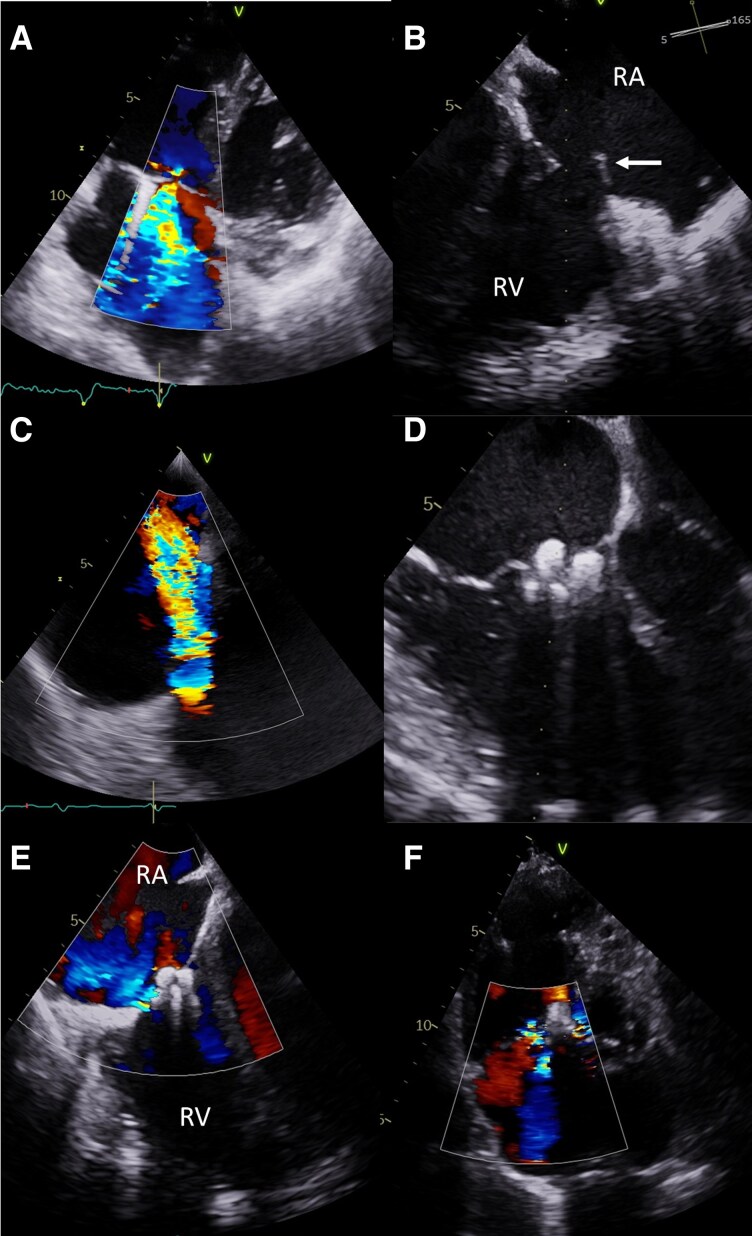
Patient 3 *(A)* Suspected conflict between the ventricular lead and the anterior tricuspid leaflet, causing significant TR. *(B)* Flail of the anterior leaflet of TV marked by the arrow after TLE. *(C)* Intra-procedural echocardiography during TLE documenting progression of TR to torrential grade. *(E)* Tricuspid valve after implantation of two PASCAL Ace devices. *(F)* Reduction of TR to moderate, maintained at follow-up visit. RA—right atrium; RV—right ventricle.
